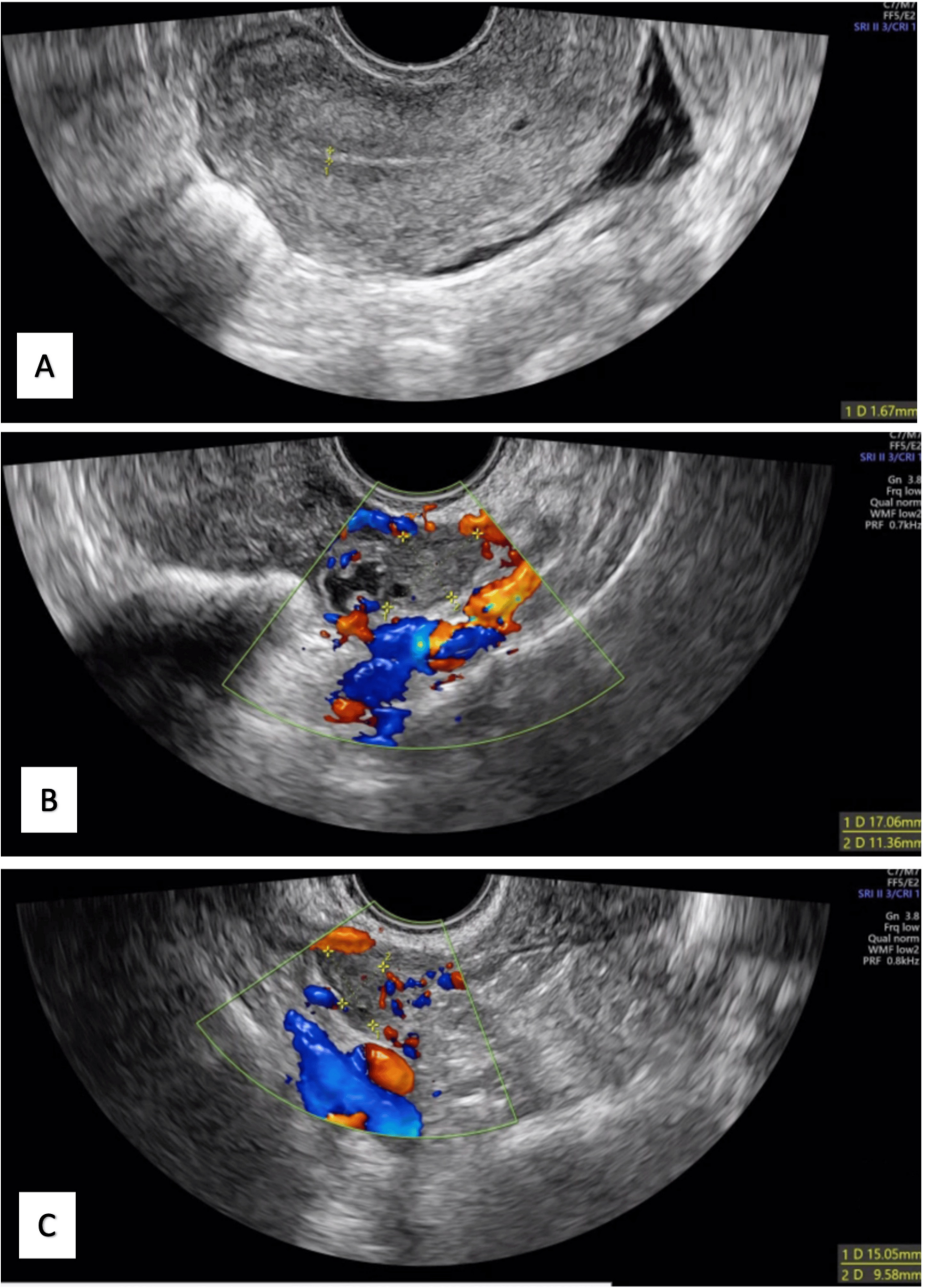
Transvaginal ultrasound revaluation one year after treatment with antituberculous drugs (A) Uterus with a thin and regular endometrium, and a thin layer of free fluid in the pouch of Douglas. (B) Right adnexal area with a normal ovary and mild hydrosalpinx. (C) Left adnexal area, with the left ovary and without the anechoic multiloculated cystic lesions previously described.
